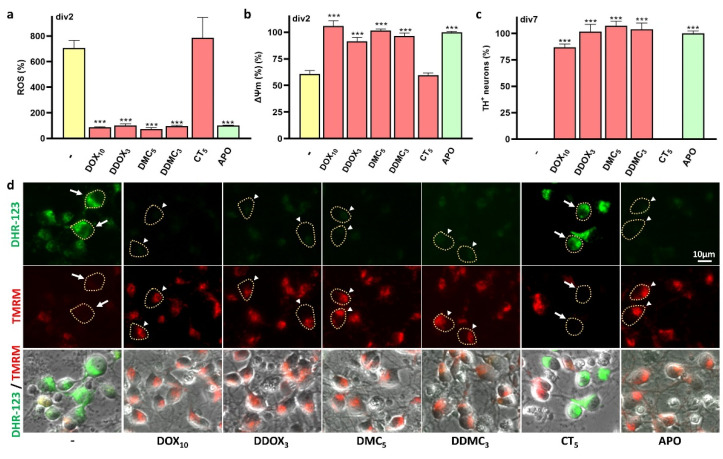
DOX, DDOX, DMC, and DDMC limit oxidative stress and preserve mitochondrial membrane potential (ΔΨm). (a,b) ROS production and changes in ΔΨm monitored with the fluorogenic probes DHR-123 and TMRM, respectively, in div2 midbrain neurons exposed or not exposed to DOX (10 µM), DDOX (3 µM), DMC (5 µM), DDMC (3 µM), or CT (5 µM). APO (100 µg/mL) was used as a reference neuroprotective treatment. Data expressed in % of APO-treated cultures are means ± SEM (4–12). * *p* < 0.001 vs. NT. One-way ANOVA followed by Dunnett’s test. (c) Survival-promoting effects of treatments described in (a,b). Data expressed in % of APO-treated cultures are means ± SEM (4–16). p* < 0.001 vs. NT. One-way ANOVA followed by Dunnett’s test. (d) Images showing DHR-123 (upper panel) and TMRM (mid panel) fluorescent signals in div2 midbrain cultures treated or not treated with DOX, DDOX, DMC, DDMC, or CT. The lower panel represents Phaco images merged with the DHR-123 and TMRM fluorescent signals. White arrows point to neurons showing a decrease in ΔΨm with an increase in ROS production. White arrowheads indicate neurons, in which ΔΨm is preserved and ROS production contained. Yellow dotted lines represent virtual boundaries of neuronal cell bodies indicated by white arrows or white arrowheads.
